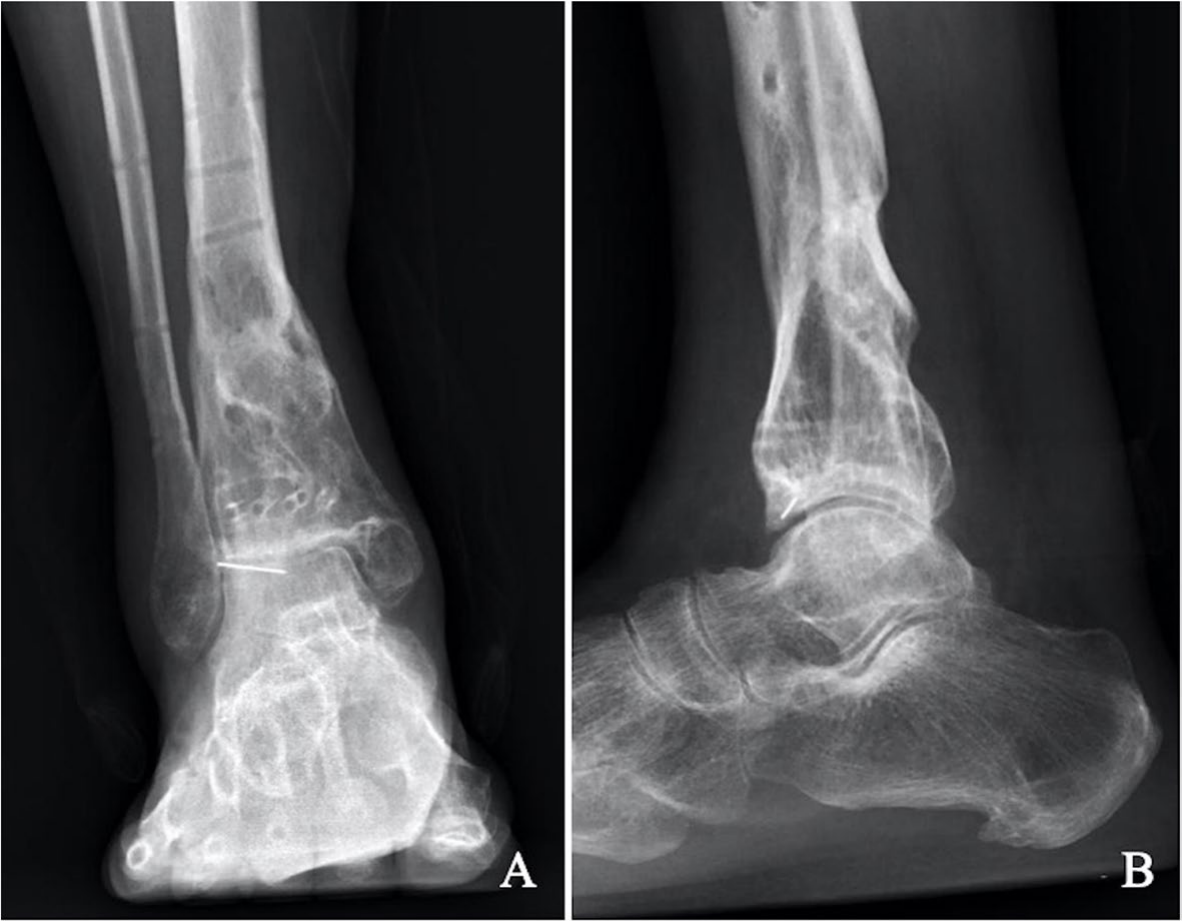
At the 20-month follow-up, plain radiographs show bone healing and good alignment (A, B). He had returned to normal activities with complaints of mild intermittent ankle pain and stiffness
